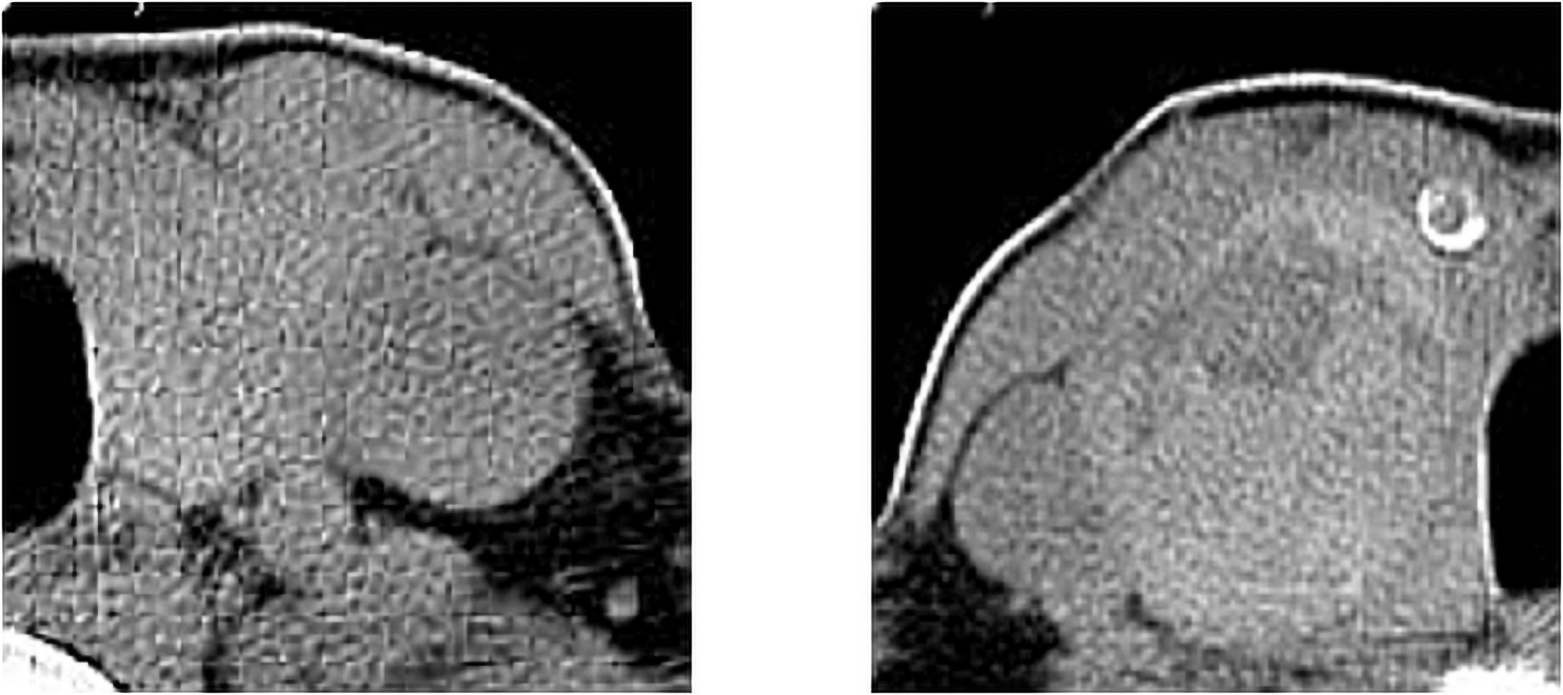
Segmented thyroid gland CT scans from patient No. 277: Left-side is Goiter (Benign) and right-side is Papillary Cancer (Malignant).
